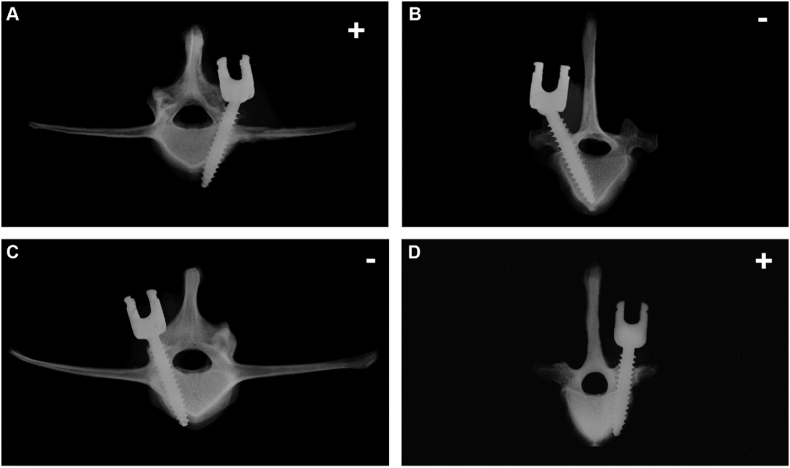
Representative cabinet x-rays of dissected vertebrae in the transverse plane. Positive control sites included are one lumbar (A) and one thoracic (D) vertebrae. Negative control sites included are one lumbar (C) and one thoracic (B) vertebrae. Positive control sites are indicated by + and negative control sites by -.
